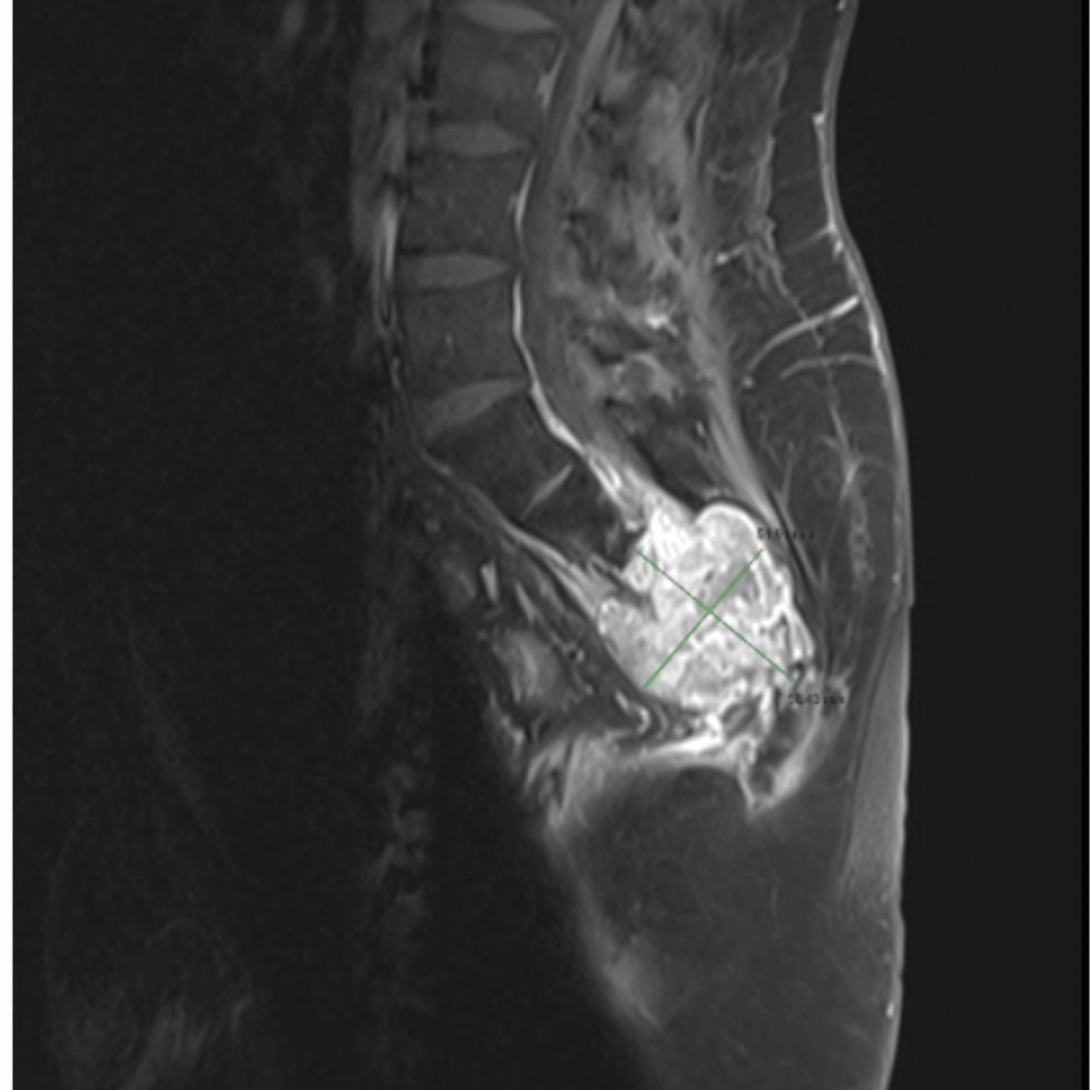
Enhanced-contrast MRI after the treatment. Sacral mass measuring approximately 5.8 cm × 5.1 cm.
